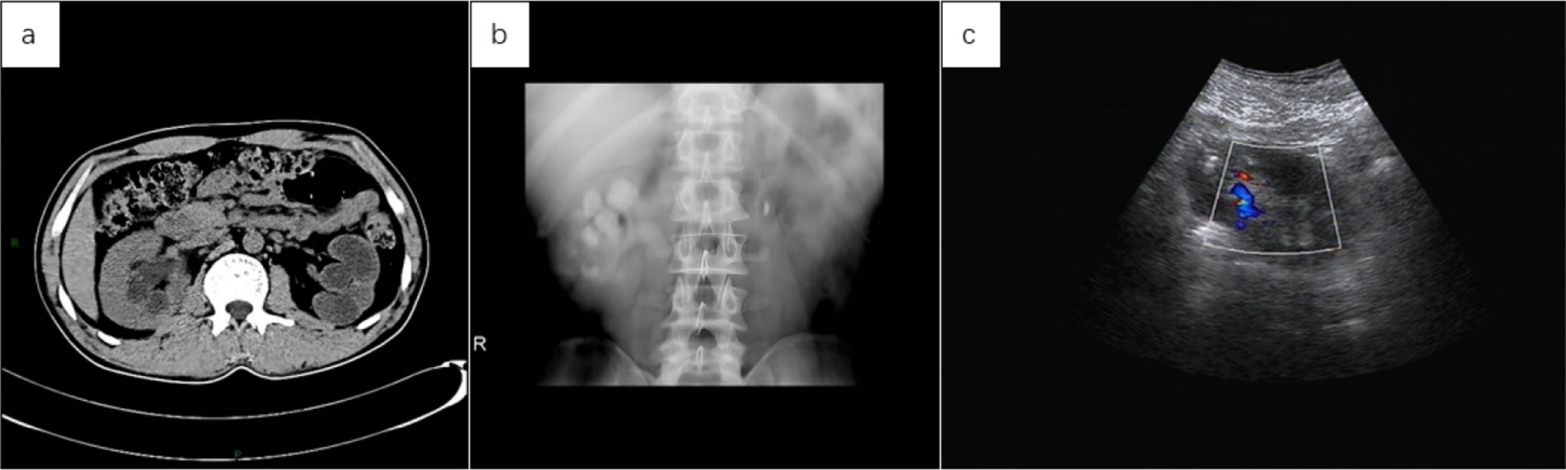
(a) the abdominal CT scan revealed a reduced left kidney with significant dilation of the renal pelvis and thinning of the renal cortex, suggesting a non-functional left kidney due to pus. The right kidney appears compensatorily enlarged, with slight dilation of the right renal pelvis. (b) Following reexamination after left nephrectomy, IVU revealed slight dilation of the right calyx and renal pelvis, no contrast filling in the left kidney, and a strip-like high-density shadow in the left hilar region, suggestive of a surgical stapler shadow. (c) Repeat examination after left nephrectomy showed no obvious abnormalities in the size of the right kidney, with slight dilation of the renal pelvis on USG.
